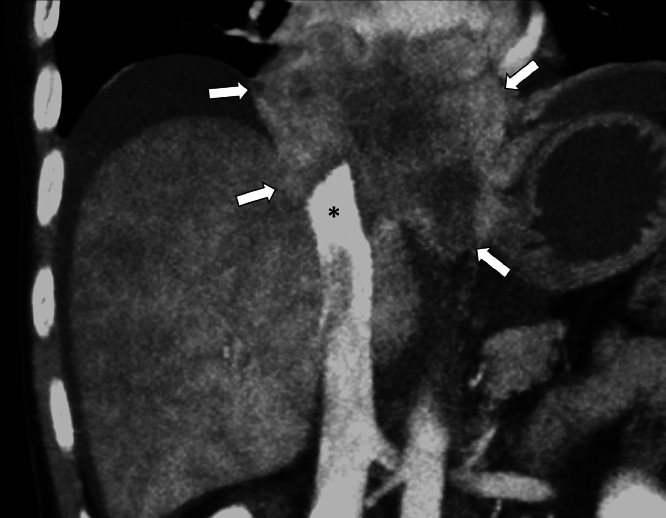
CT of mediastinal mass progression and obstruction. Coronal contrast enhanced CT, maximum intensity projection of the abdomen demonstrates a large mass (arrows) in the lower chest with acute obstruction of the IVC (*).
